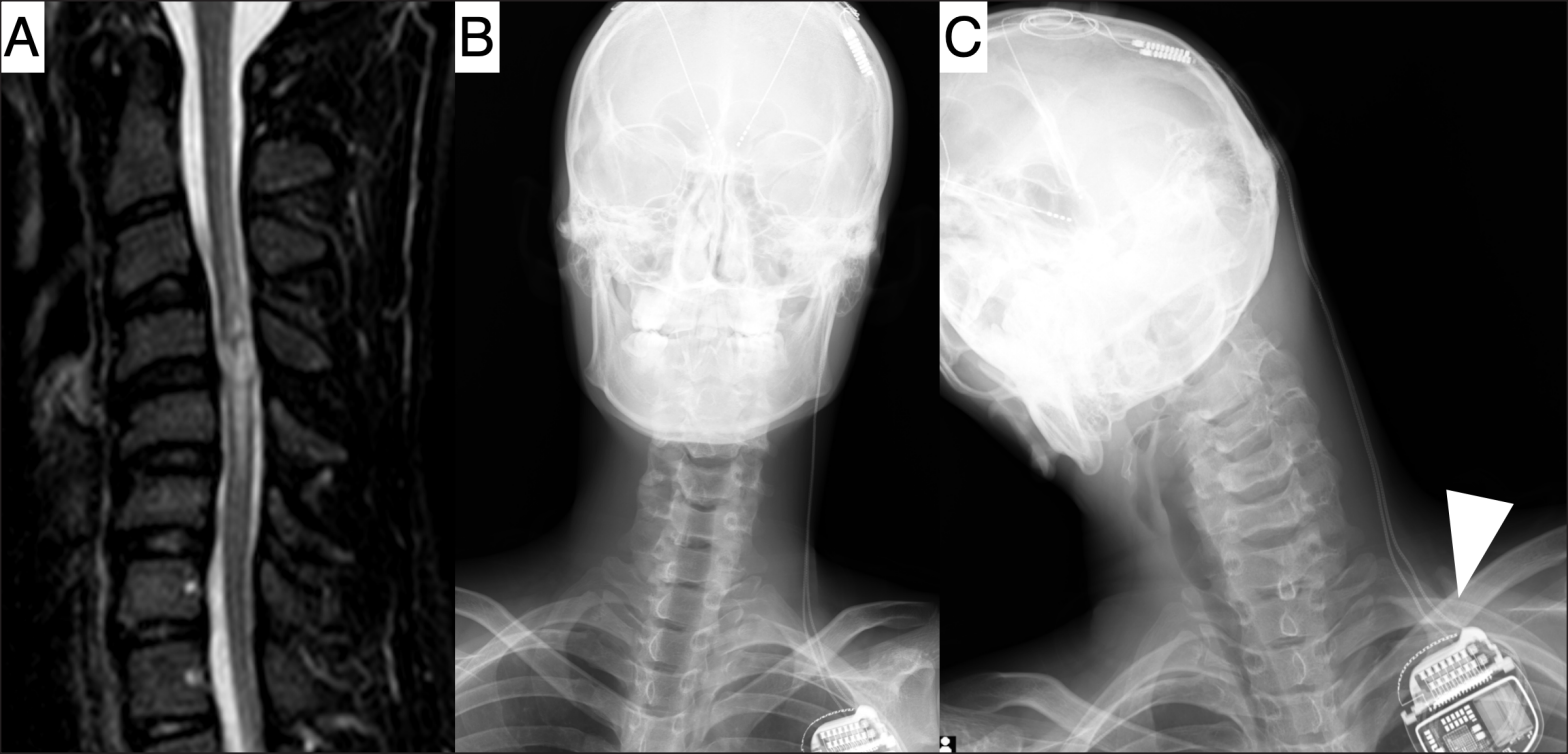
Pre- and postoperative evaluation of case 2. Sagittal T2-weighted magnetic resonance imaging revealed a high-intensity area extending from the C3 to C6 levels (A). Postoperative X-ray confirmed proper lead placement (B). When the neck was extended, the IPG caught on the clavicle, placing stress on the cable (arrowhead), which was presumed to have caused cable breakage (C).
